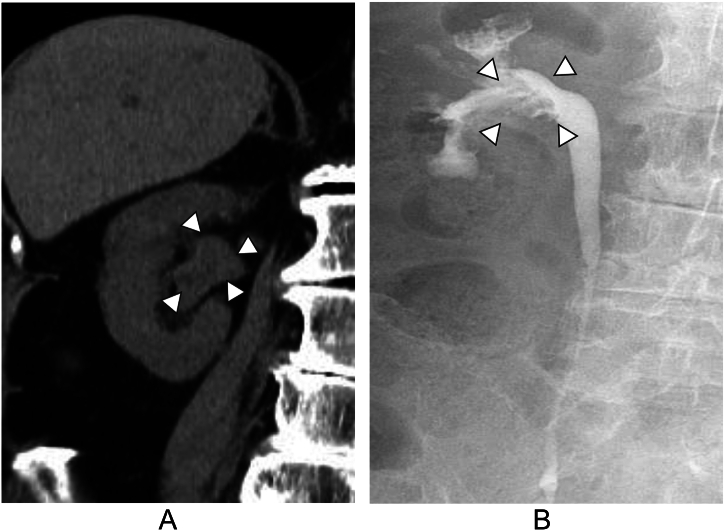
Abdominal computed tomography scan and retrograde pyelography images. (A): Abdominal computed tomography scan shows right hydronephrosis (white arrows). (B): Retrograde pyelography shows defect (white arrows) of contrast agent in the right pelvis.
